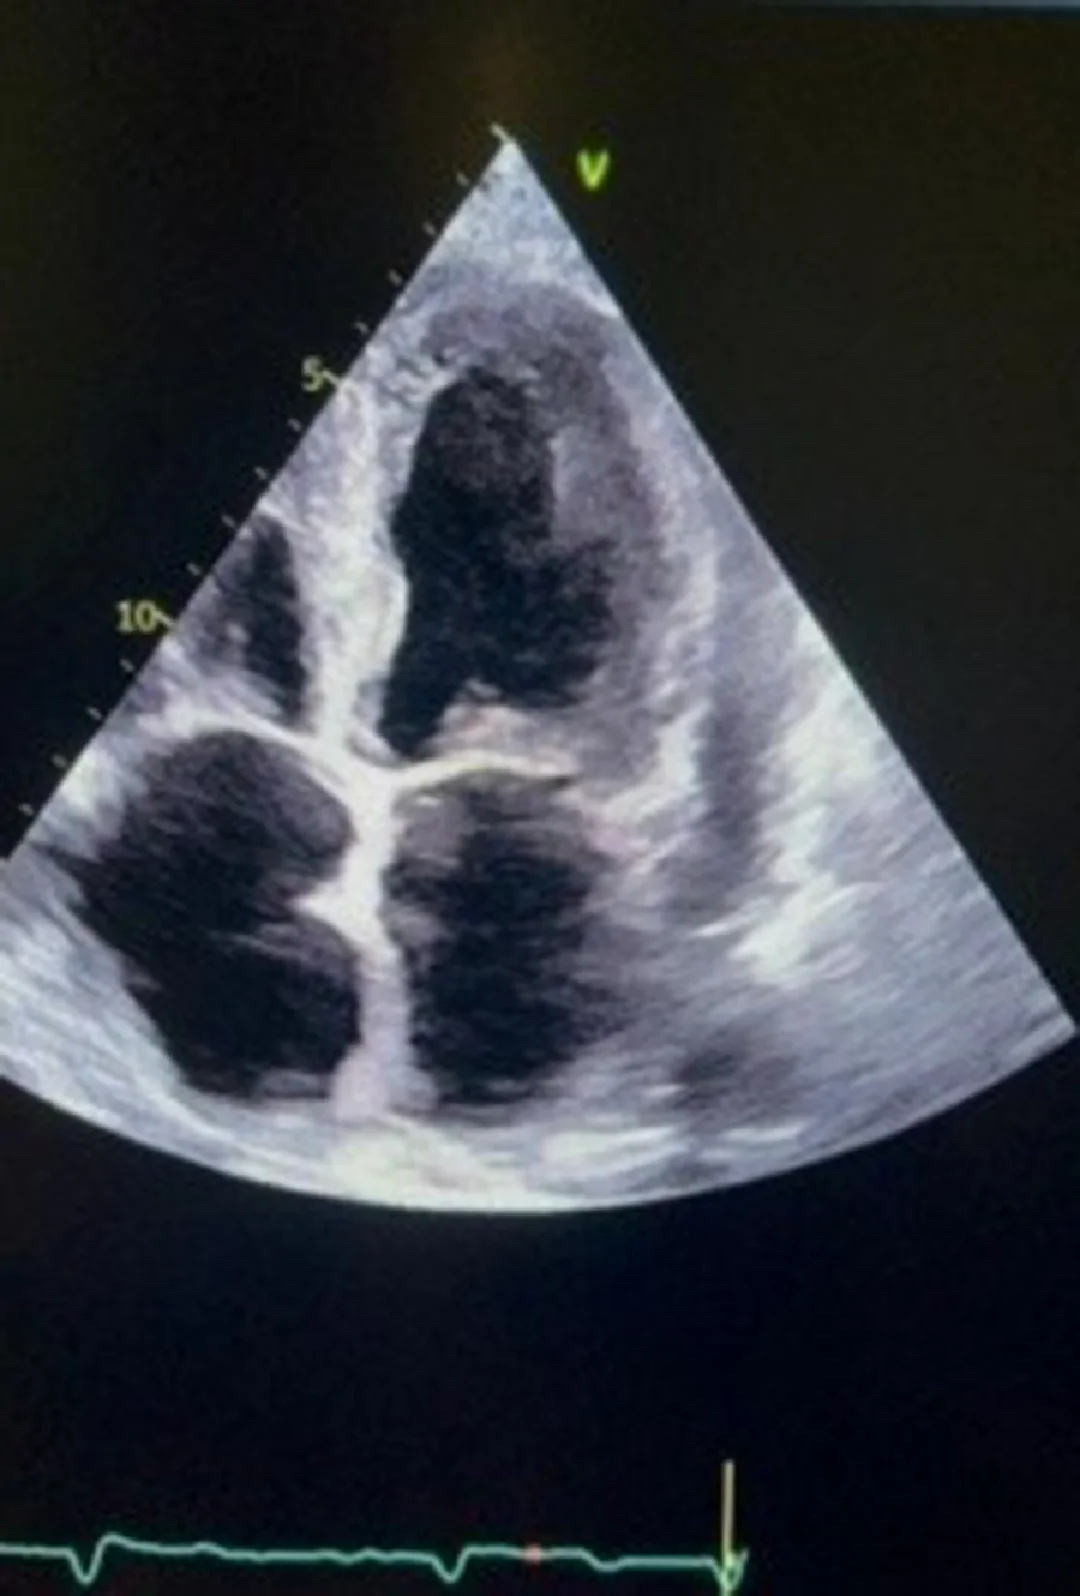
Apical 4-chamber view showing bi-atrial enlargement with ventricular hypertrophy and mild dilatation.
